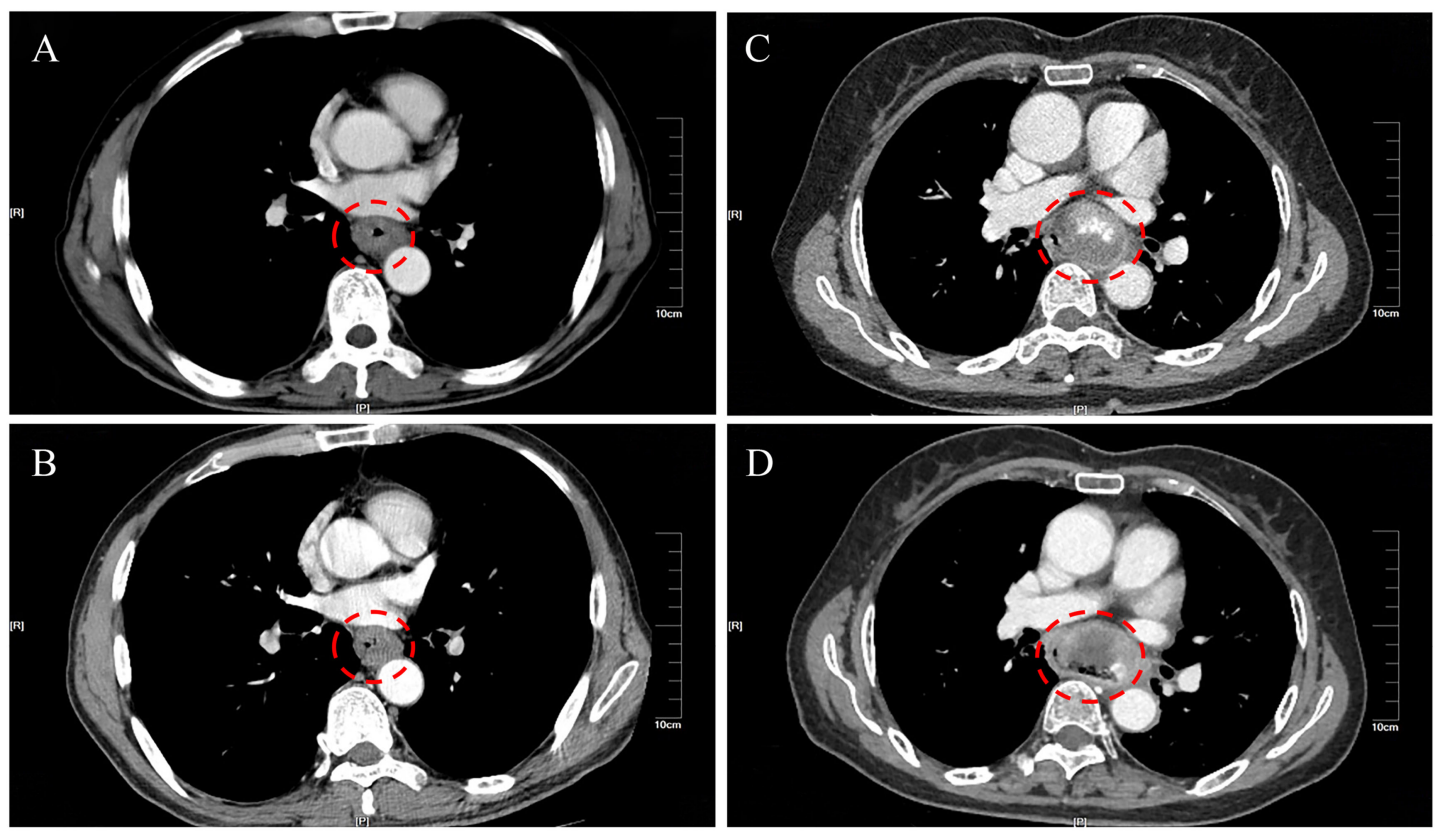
Comparison of thoracic enhanced CT scans of esophageal cancer tumors before and after neoadjuvant chemotherapy. (A) Case 3 before neoadjuvant treatment; (B) Case 3 after neoadjuvant treatment; (C) Case 10 before neoadjuvant treatment; (D) Case 10 after neoadjuvant treatment.
